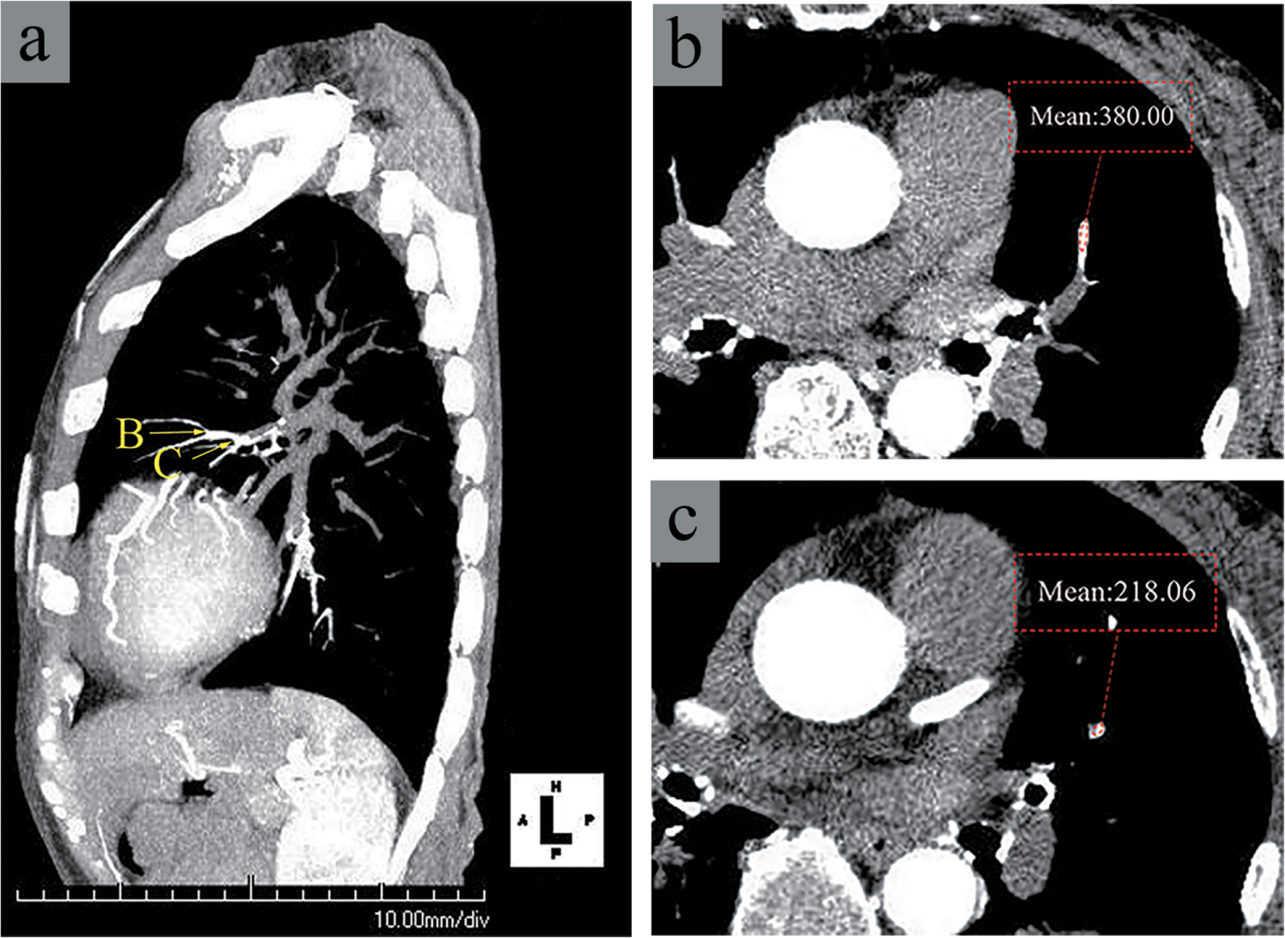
Enhanced pulmonary arteries shown on intra-aortic computer tomographic angiography in a representative patient (72-year-old woman with chronic thromboembolic pulmonary hypertension). a Sagittal view of the partial maximum projection (MIP) image with 20 mm slice thickness showing enhancement in the pulmonary artery of the left S4 by contrast media injected in the ascending aorta; arrows B and C indicate slice levels of the corresponding original transverse images shown in b and c, respectively. Transverse images at levels corresponding to arrows B and C in the image shown in a. The numbers indicate the mean CT values (HU) at regions of interest (ROIs) placed on the segmental pulmonary artery (A4). The mean of the CT values was regarded as the HU-PA for the left A4
